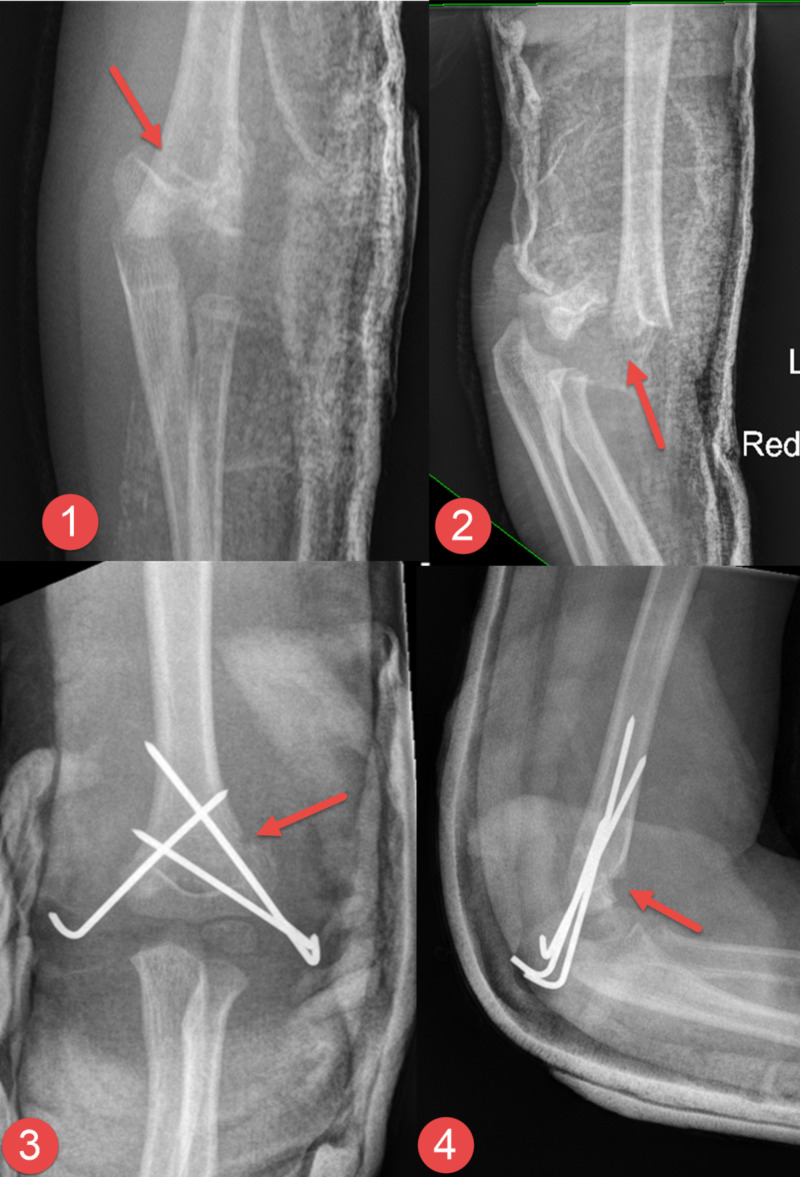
Plain radiograph of the elbow following surgery Plain radiograph of the elbow showing the pre-operative anteroposterior (AP) and lateral views (images 1 and 2, respectively) and postoperative AP and lateral views (images 3 and 4, respectively). The fracture (red arrows) was reduced and stabilized with three K-wires - two were inserted laterally and one was inserted medially.
